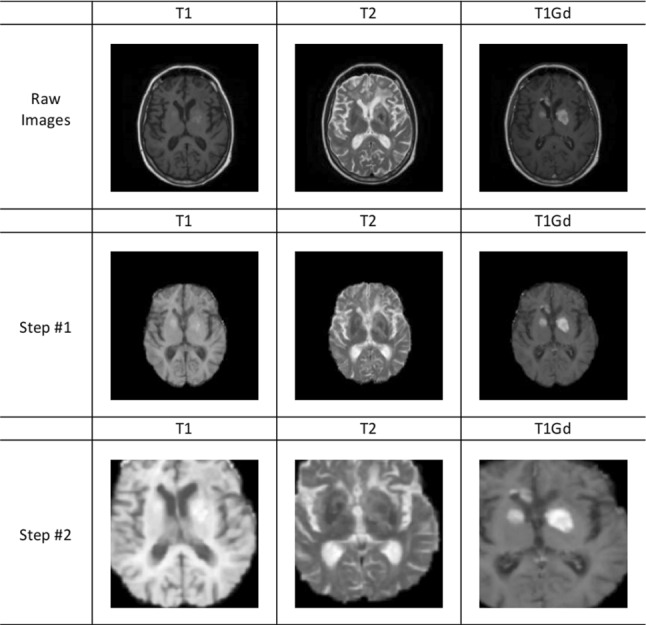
Preprocessing steps of MRI of patients with PCNSL
